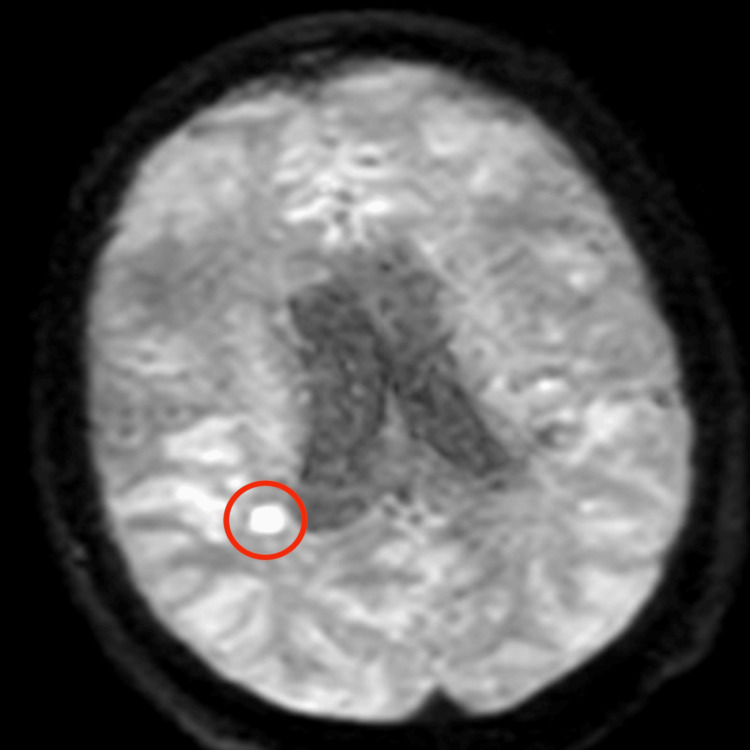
Axial diffusion-weighted (DW) MRI demonstrating a focal hyperintense lesion in the right posterior frontal cortical-subcortical region, adjacent to the insular cortex, consistent with acute ischemic infarction (red circle indicates the area of restricted diffusion). MRI: magnetic resonance imaging
